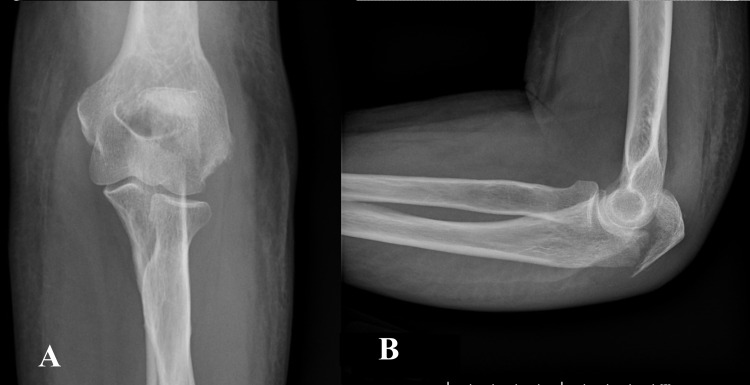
Plain radiographs of the injured left elbow. Anteroposterior (A) and lateral (B) radiographs of the injured elbow showing a displaced long oblique olecranon fracture along with cortical thinning and generalized increased bone radiolucency.
